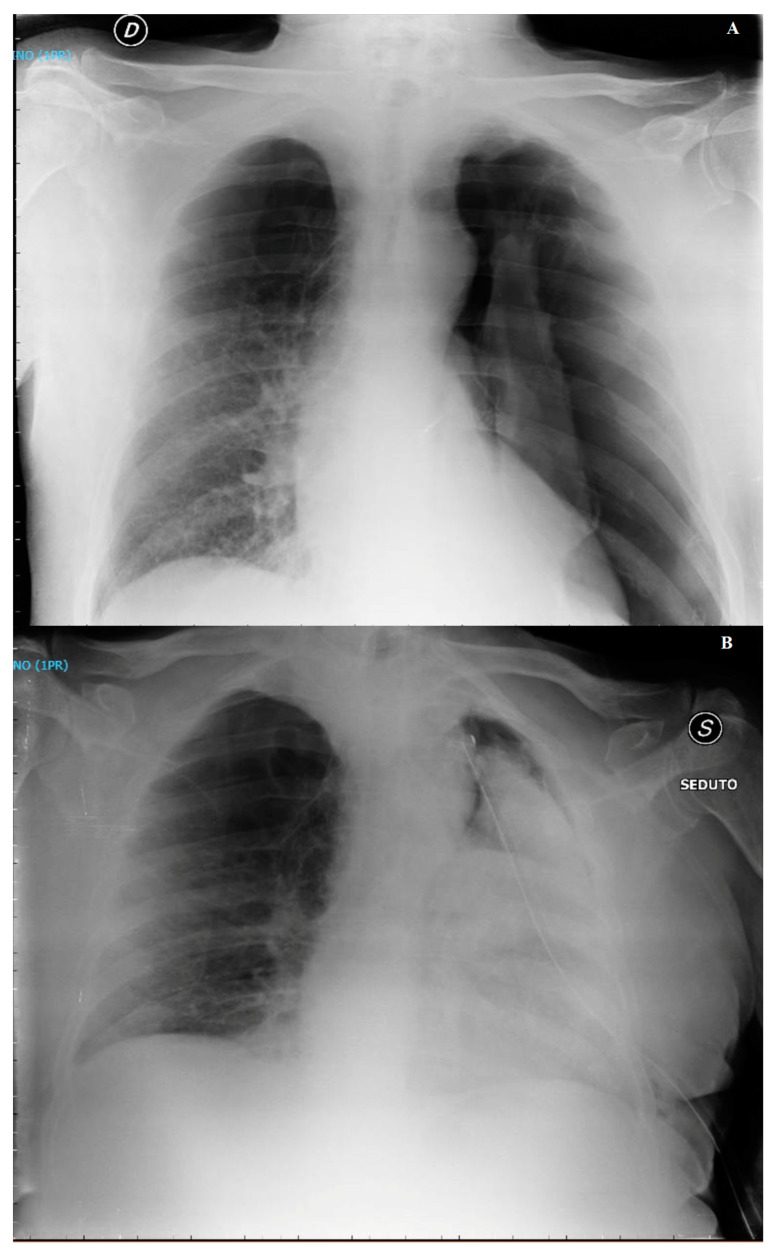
(A) Chest X-ray, left massive pneumothorax; (B) chest X-ray, left re-expansion edema 6 h after chest tube positioning.
